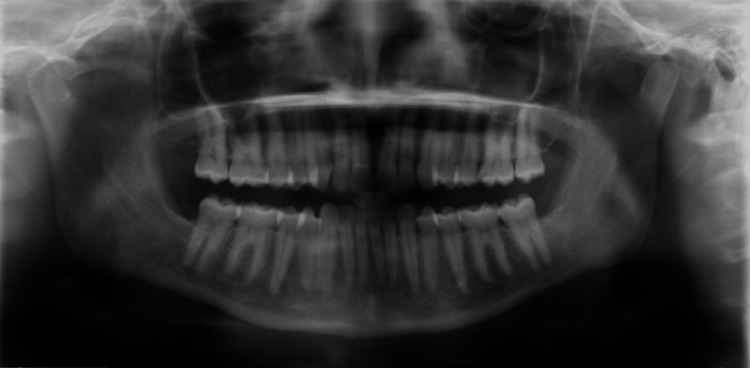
An orthopantomogram performed six months after the extraction of all wisdom teeth
